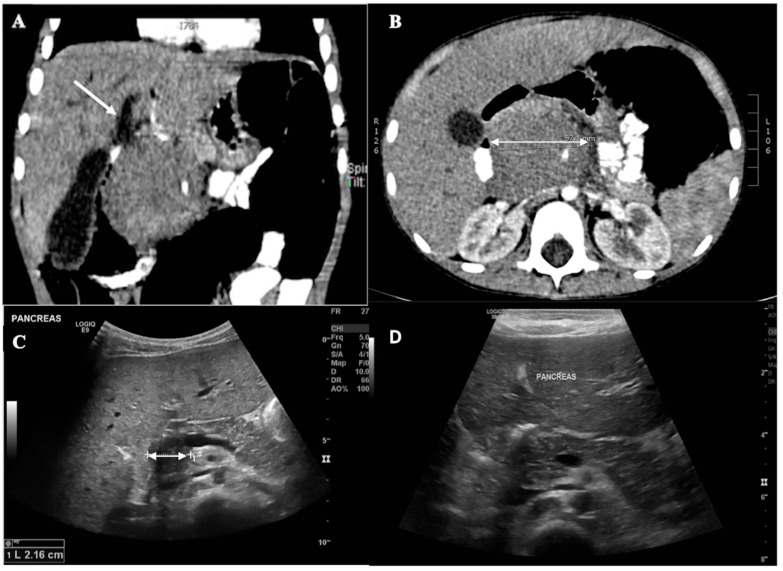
(A,B): Axial and coronal contrast enhanced CT images of the abdomen show a well-defined large round homogenous mass at the region of the pancreatic head measuring 5.2 cm × 5.7 cm × 6.1 cm displacing adjacent structures and encasing superior mesenteric artery, with upstream biliary tree dilatation (arrow). (C): Greyscale axial ultrasound image at the level of pancreatic head after starting chemotherapy obtained on day 17 shows mass size reduction measuring 3.4 cm × 3 cm × 2 cm, with reduced upstream biliary tree dilatation. (D): Grey scale ultrasound image after induction 2 showed no songoraphic residual mass, with resolved biliary tree dilatation.
